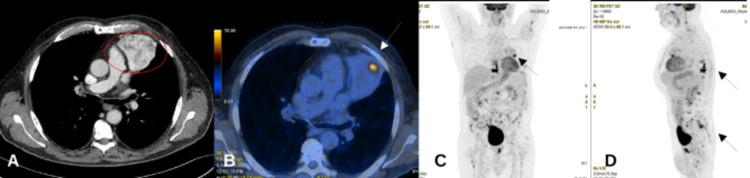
Imaging of the mediastinal neoplasm at CT scan and at FDG-PET scan The mediastinal mass of 81x73x54mm (red circle) at CT-scan (A). FDG-PET scan: a focal hypermetabolism with SUVmax 11.30 (white arrow) within negative surrounding tissue (B). Advanced prostatic adenocarcinoma with multiple metastases at the thoracic vertebrae, femur, sacrum and within the mediastinal lesion (black arrows) (C, D).
